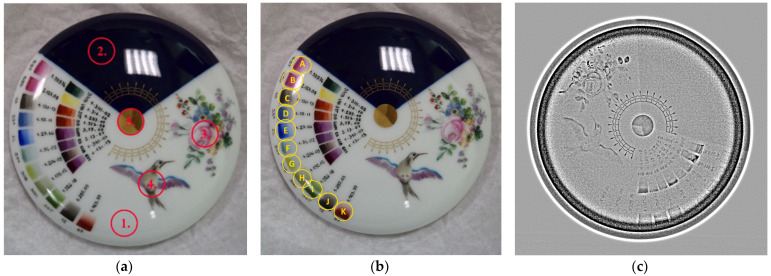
(a) PGAA measurement spots (1–5) selected for decoration, (b) color palette (A–K), and (c) dual-energy X-ray radiogram.
